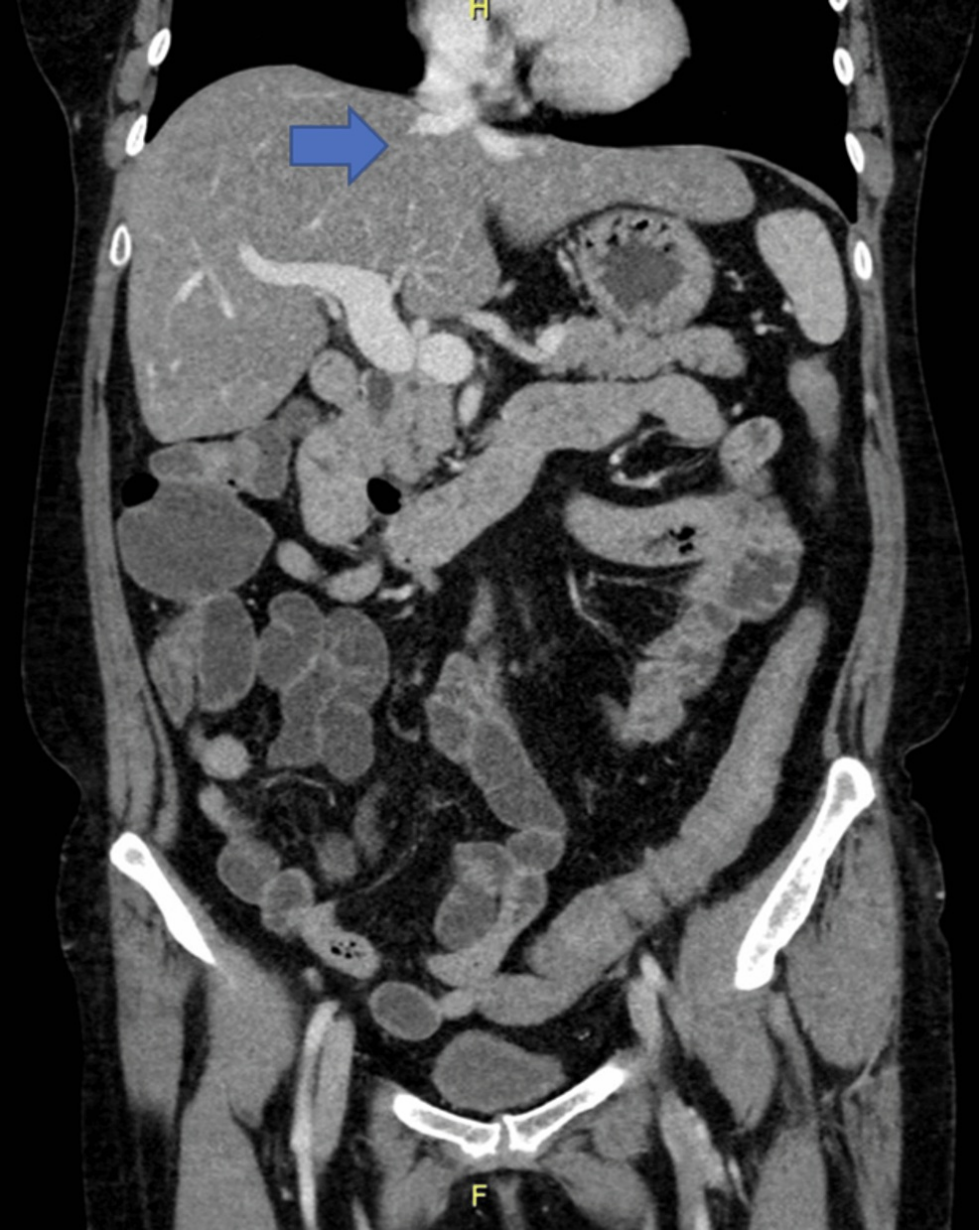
CT abdomen and pelvis, coronal reconstruction, shows the diminutive right and left hepatic veins draining into the suprahepatic inferior vena cava remnant (blue arrow)
